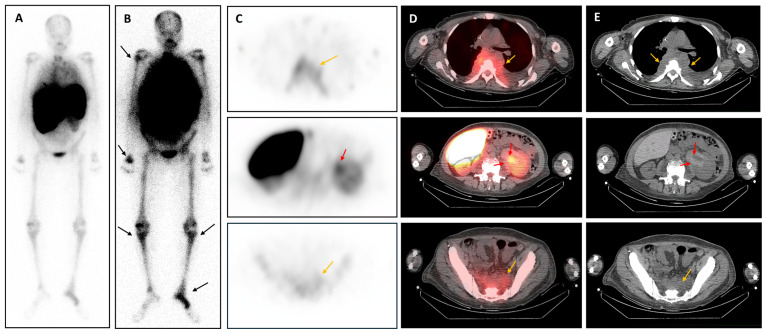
[^99^mTc]-sulphur colloid scintigraphy with SPECT/CT. (A) Whole-body planar anterior image. (B) Corresponding anterior planar image displayed with increased intensity scaling to better depict the heterogeneous skeletal tracer distribution, most evident in the peripheral skeleton and long bones (black arrows), consistent with redistributed active haematopoiesis. (C) Axial SPECT images showing tracer uptake in the thoracic paravertebral lesion (orange arrow, upper panel), left peripelvic/pelvicalyceal lesion (red arrow, middle panel), and presacral lesion (orange arrow, lower panel). (D) Corresponding fused SPECT/CT images. (E) Corresponding axial CT images. As tissue sampling was considered unsafe because of the high haemorrhagic risk in this cytopenic patient, [^99m^Tc]-sulphur colloid scintigraphy with SPECT/CT was performed to support a marrow-related aetiology (Figure 3). This demonstrated concordant tracer uptake in the paravertebral, presacral, and left peripelvic lesions, strongly supporting multifocal extramedullary haematopoiesis (EMH). Initial management consisted of supportive care, including platelet transfusions. She was then treated with ruxolitinib for 3 months as bridging therapy before allogeneic haematopoietic stem cell transplantation, with subsequent favourable clinical and biological improvement over the following 6–8 months. Extramedullary haematopoiesis (EMH) is a compensatory process in which haematopoiesis occurs outside the bone marrow when normal marrow function becomes ineffective, most commonly in chronic anaemias and myeloid neoplasms such as myelofibrosis [1,2]. Although previously described, renal EMH remains rare, and its imaging appearance may still pose a significant diagnostic challenge [1,2,3,4,5,6,7]. Reported CT appearances of renal EMH include perinephric plaques, hilar or perihilar masses, and infiltrative soft tissue centred on the renal sinus and pelvicalyceal system, sometimes extending to the proximal ureter and causing obstruction or renal dysfunction [1,2,3,4,5,6,7]. In our patient, the principal differential diagnoses were upper tract urothelial carcinoma, infiltrative haematological disease such as lymphoma or leukaemic/myeloid infiltration, and, less likely, other infiltrative inflammatory or infectious processes [3,4,5,6,7].
